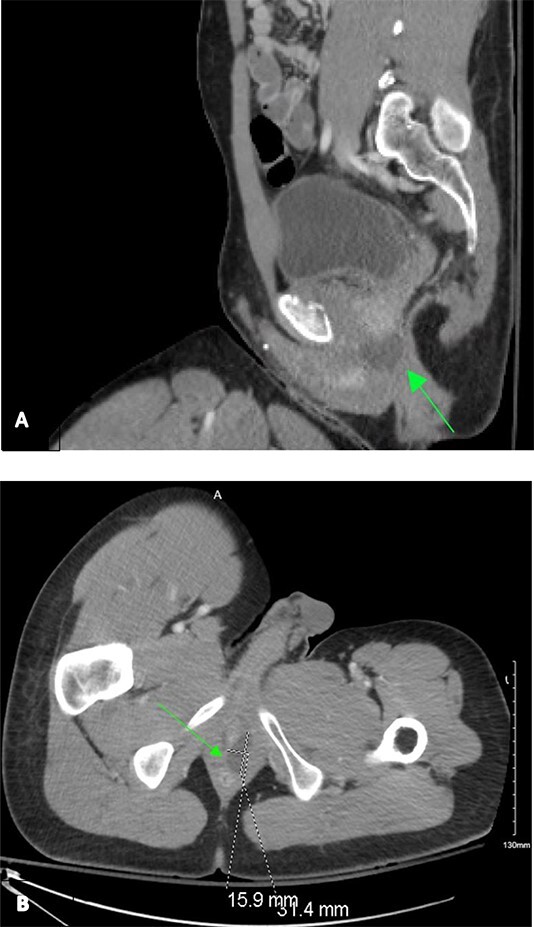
Contrast-enhanced CT chest/abdomen/pelvis during a tier 2 trauma activation, revealing a homogenous hypodense perianal fluid collection from sagittal (A) and transverse (B) planes.
